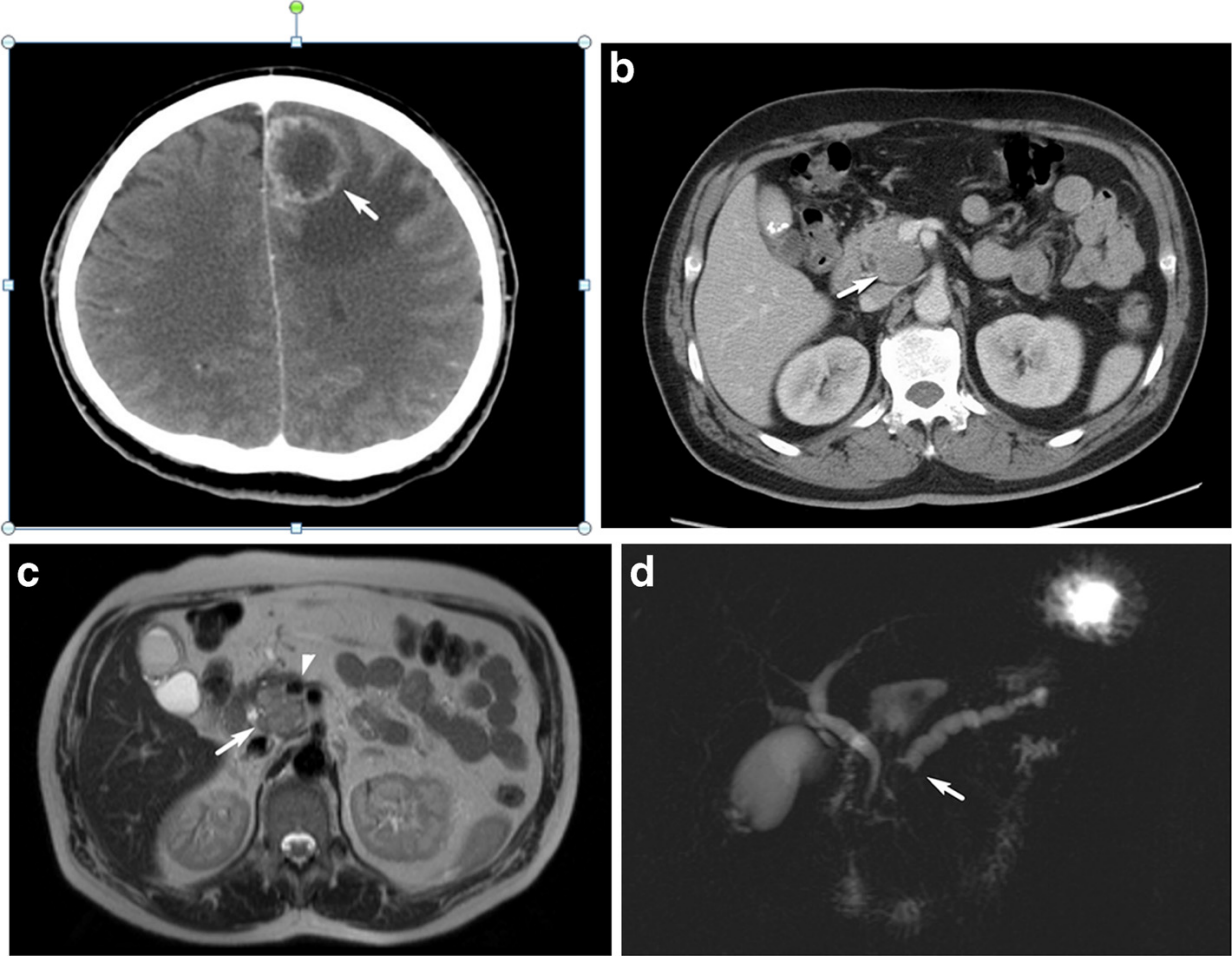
Computed tomography (CT) and magnetic resonance (MR) imaging. (a) Axial contrast-enhanced computed tomography of the brain shows a ring-enhancing lesion (arrow) in the left frontal lobe with perifocal edema. (b) Axial contrast-enhanced CT of abdomen shows a hypodense lesion (arrow) in the uncinate process of the pancreas with minimal encasement of the superior mesenteric vein. (c) 1 C T2-weighted image shows a mildly hyperintense mass (arrow) in the uncinate process of the pancreas with minimal encasement of the superior mesenteric vein (arrow head). (d) 1D MR cholangiopancreatography image shows dilatation of the pancreatic duct with abrupt termination (arrow). No dilatation of the biliary tree is noted.
